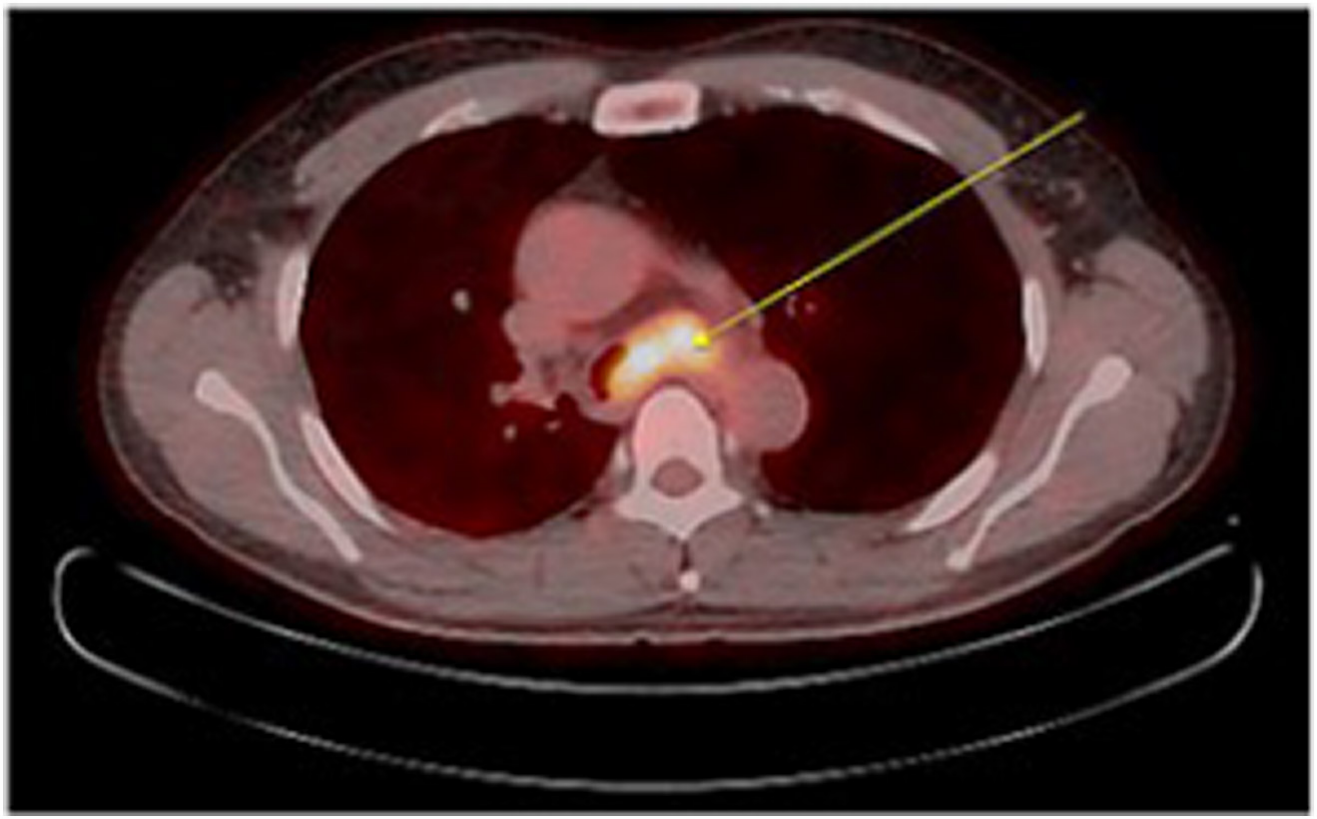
Pretreatment positron emission tomography / computed scanning demonstrating a hypermetabolic lesion (SUVmax. 4.3) in the distal to carina level left main bronchus (arrow)—axial view.
